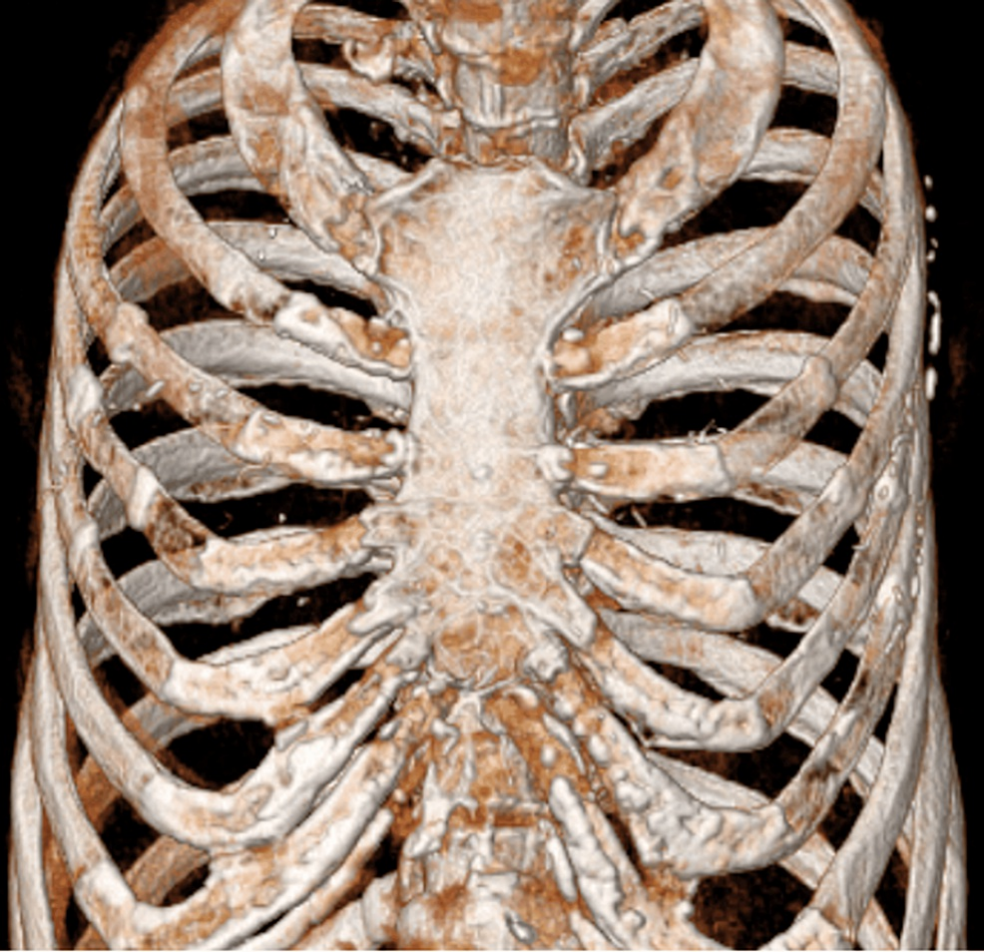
Computed tomography showing no apparent sternal fractures.
